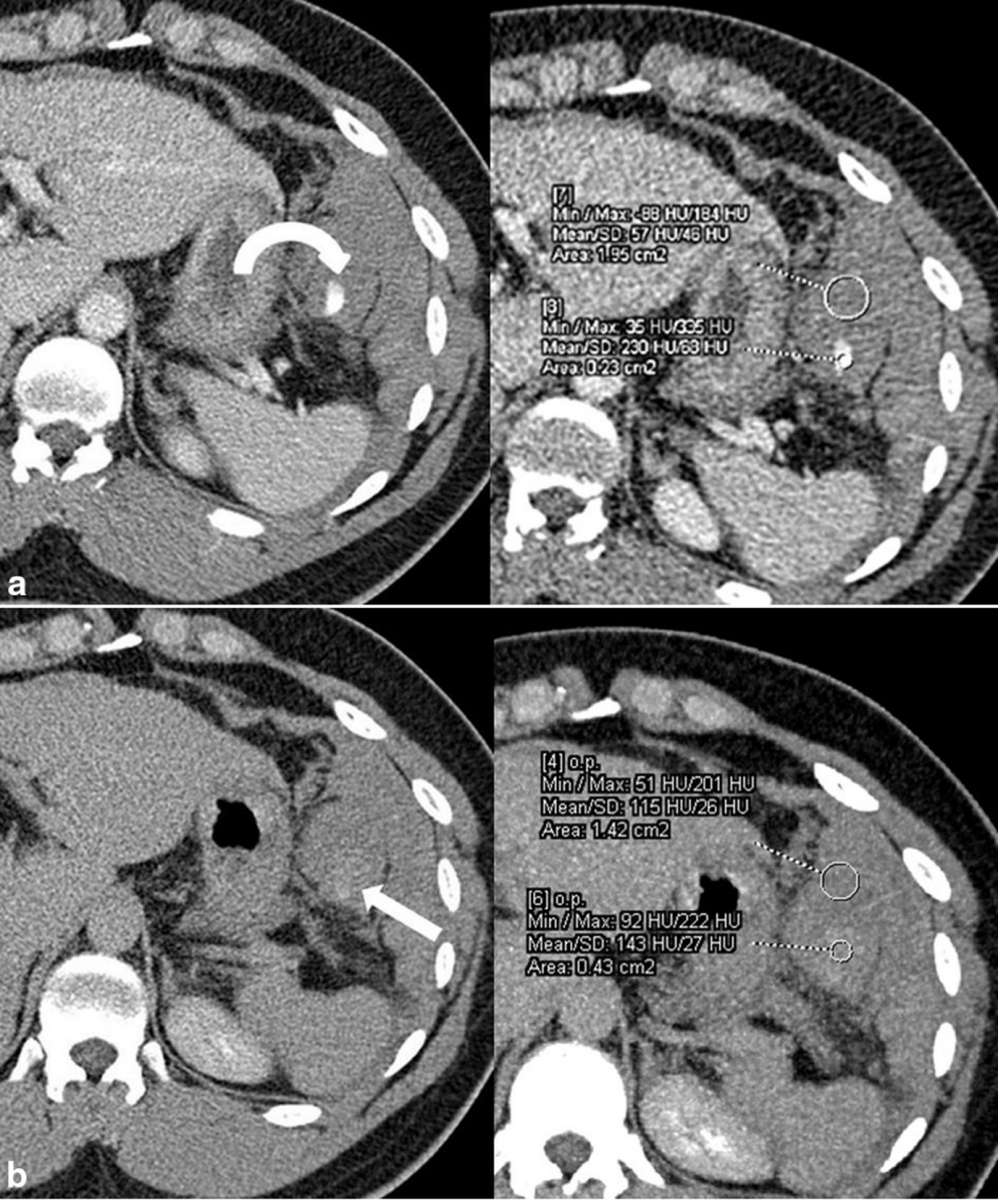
(a) Axial contrast-enhanced CT images of the upper abdomen in portal venous phase show a haematoma in the left hypochondriac region with active contrast extravasation (curved arrow). (b) Axial images in delayed phase show contrast pooling as an area of increased attenuation (arrow) compared with the haematoma.
